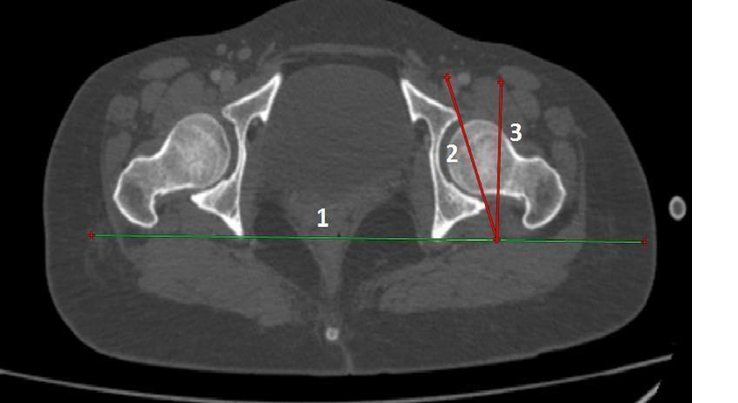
Axial computed tomography image of bilateral hip joint showing method for measurement of acetabular version (Line 1: Line connecting bilateral posterior ischia, Line 2: Line connecting anterior and posterior acetabular margins, Line 3: Line drawn perpendicular to line 1 from point of intersection of line 1 and 2: Acetabular version: Angle between line 2 and 3).
